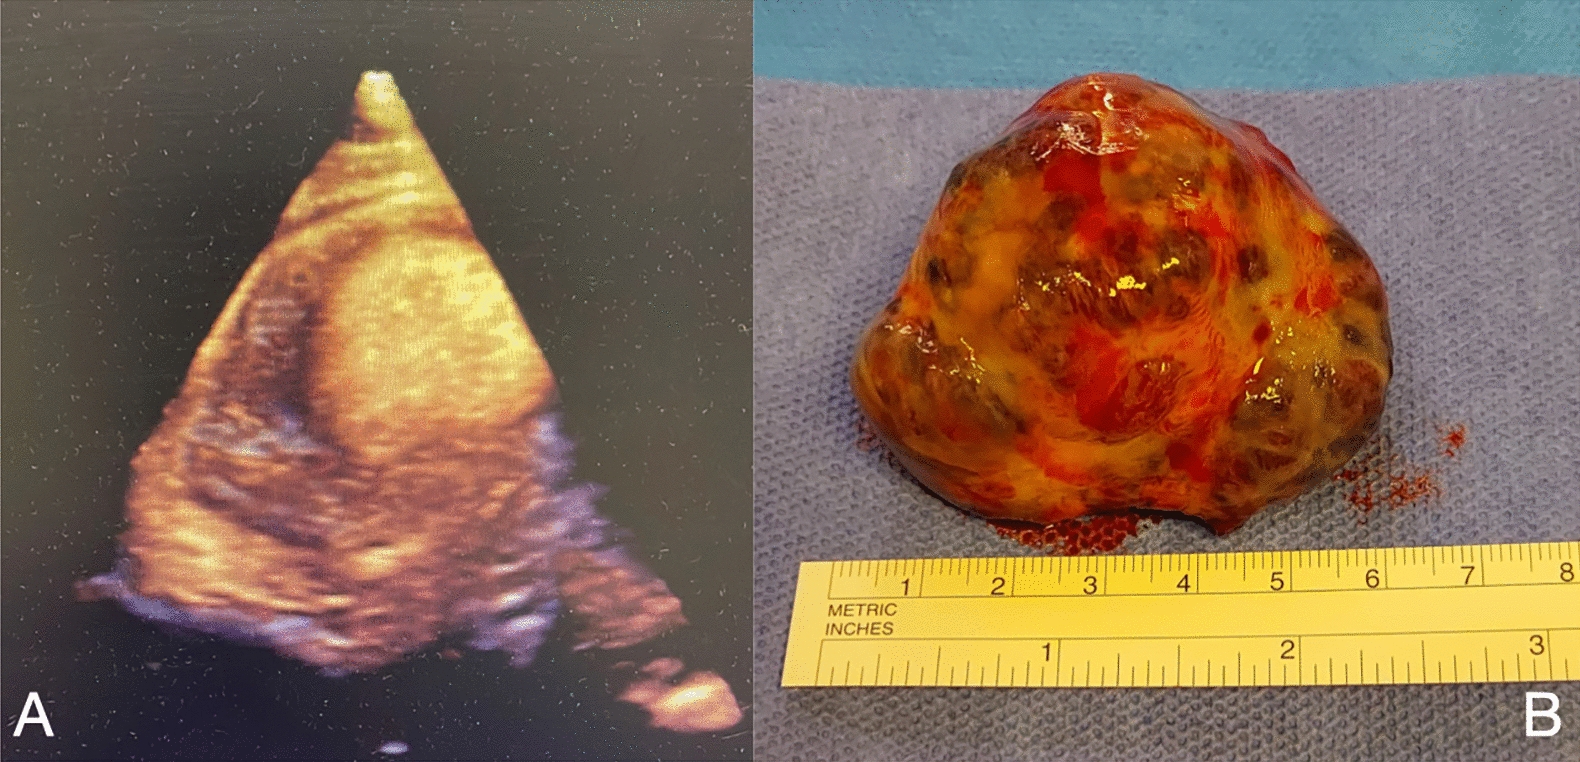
Mass dimensions A 3d echocardiography B Mass dimensions after the surgical resection (6 centimeters)
